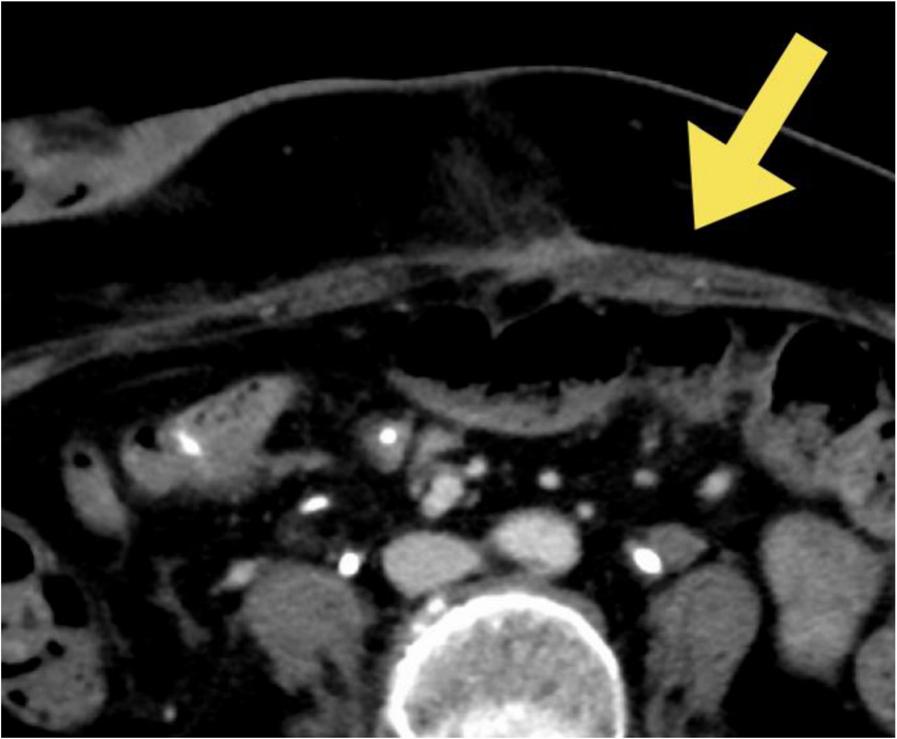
Enhanced abdominal CT on POD 16. The figure shows an enlarged left rectus abdominis with inhomogeneous contrast
